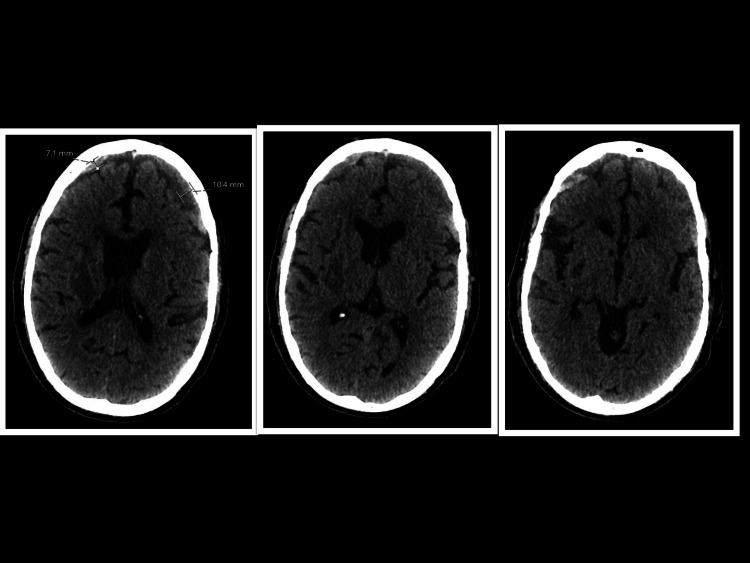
Non-contrast axial CT scan of the brain - week 8 Bilateral subdural hematomas (SDH) over the frontal lobes, right measuring 7.1 mm and left measuring 10.4 mm, with varying intensities, predominantly isodense - on a background of resolving right intraparenchymal hemorrhage (IPH).
